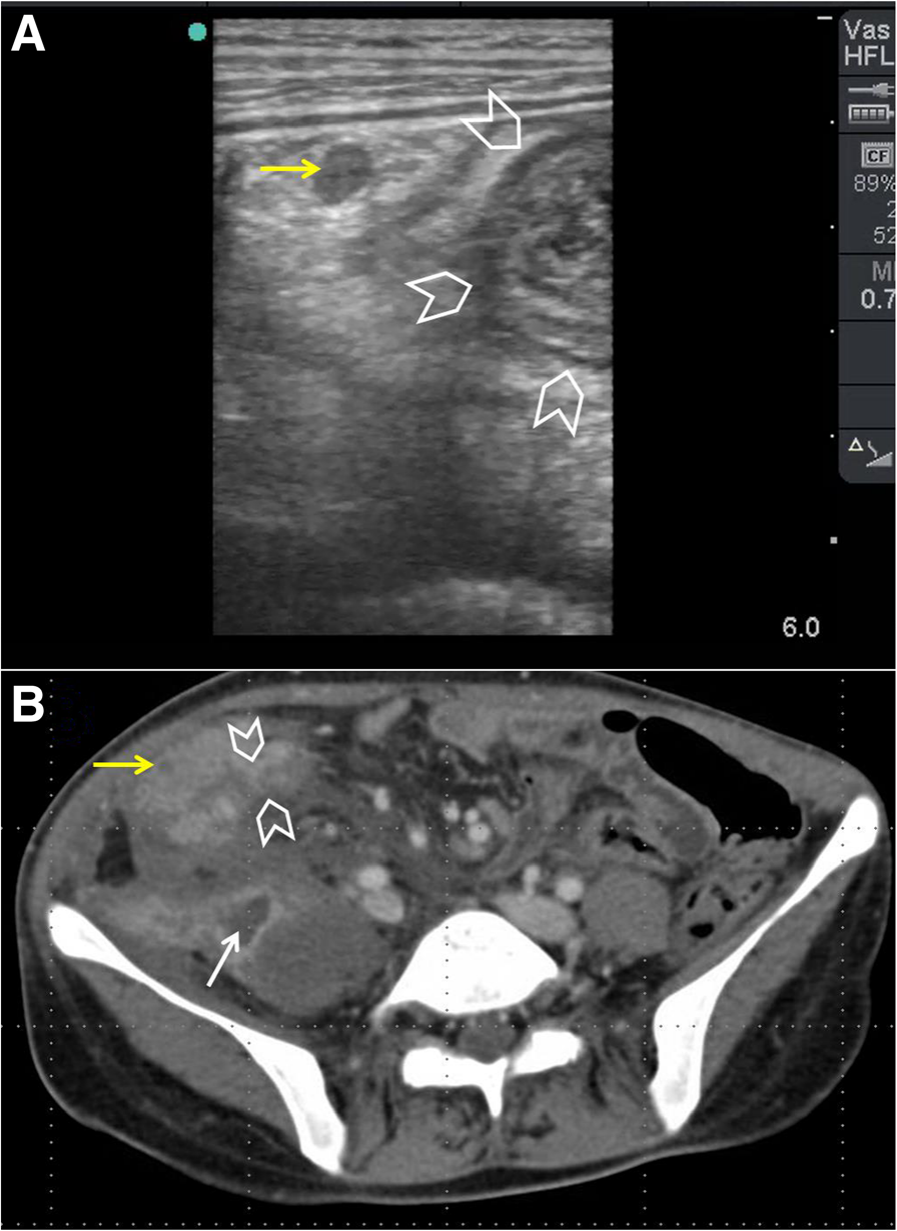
A 30-year-old woman presented with a tender right iliac fossa mass of 4 months duration. The mass was fixed and hard. POCUS (a) using a high-frequency linear probe showed matted thickened inflamed small bowel (arrowheads) and regional lymph nodes (yellow arrow). Abdominal CT scan with intravenous contrast (b) confirmed the presence of the thickened bowel (arrowheads) and the enlarged lymph nodes (yellow arrow). There was an ileo-psoas abscess (white arrow). Biopsies through a colonoscopy and an interferon test were non-conclusive. The patient was started on anti-tuberculous treatment as a therapeutic diagnosis
